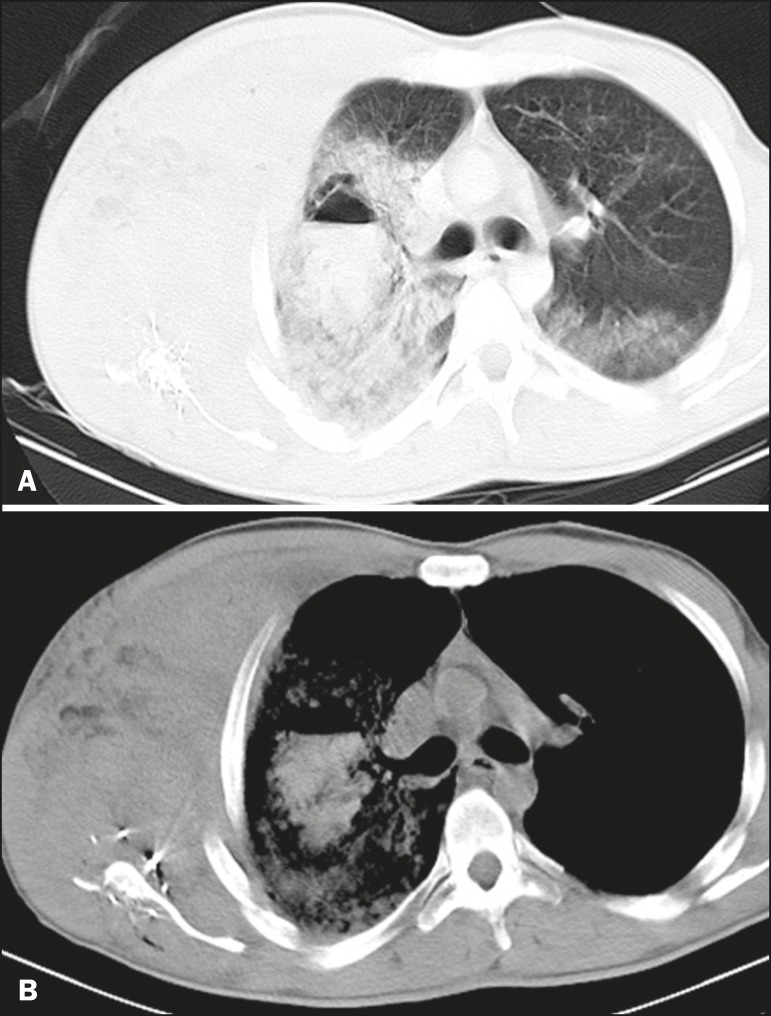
A 35-year-old male gunshot victim. Axial CT scans of the chest with a
